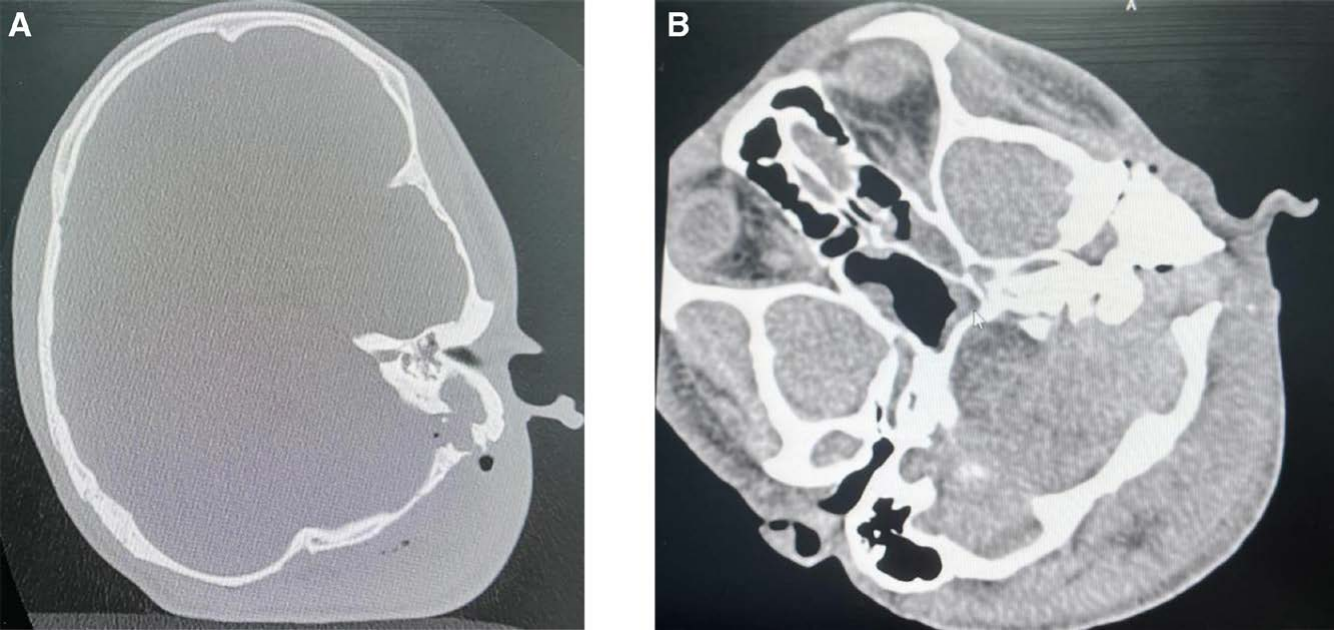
Preoperative and postoperative imaging of middle ear cholesteatoma: (A) preoperative CT image showing the presence of middle ear cholesteatoma in the left mastoid region. (B) Postoperative CT image showing the resolution of the cholesteatoma.
